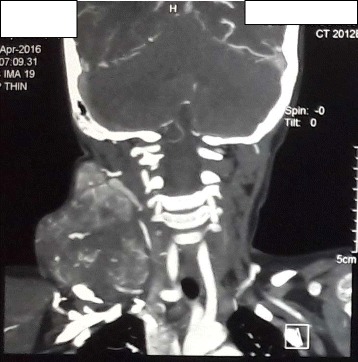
CT of neck (coronal view)
